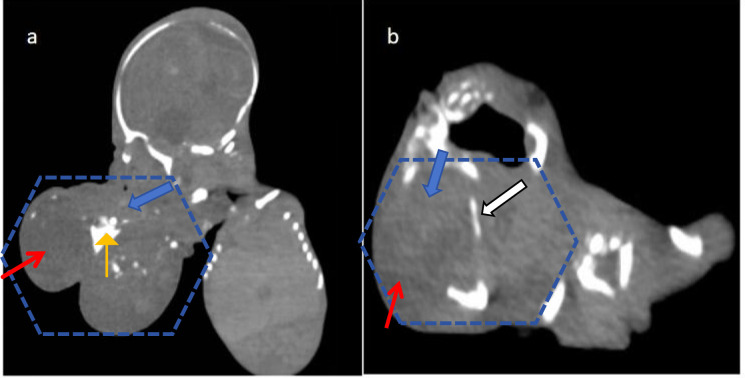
Postnatal CT findings of fetal oral immature teratoma. (a) Sagittal view shows a large mass (dashed outline) measuring 6.1 × 6.1 × 6.8 cm within the oral cavity, with broad-based attachment to the skull base, palate, and mandible. The tumor demonstrates heterogeneous density with mixed solid (S, blue arrows) and cystic (C, red arrows) components. Punctate calcifications (orange arrows) are visible within the solid portion, a feature more commonly associated with immature teratomas compared to mature counterparts. The mass causes significant oropharyngeal narrowing, indicating airway compromise. Vascularity: Non-contrast CT cannot directly assess tumor blood supply; however, the solid component shows homogeneous soft-tissue density without visible intratumoral vessels, a pattern consistent with moderately vascular immature teratomas. (b) Transverse view reveals erosion and destruction of the mandibular bone (white arrows), reflecting the locally invasive behavior characteristic of high-grade immature teratoma. The tumor mass is outlined by a dashed contour, with mixed solid (S, blue arrows) and cystic (C, red arrows) components. S, solid component; C, cystic component.
